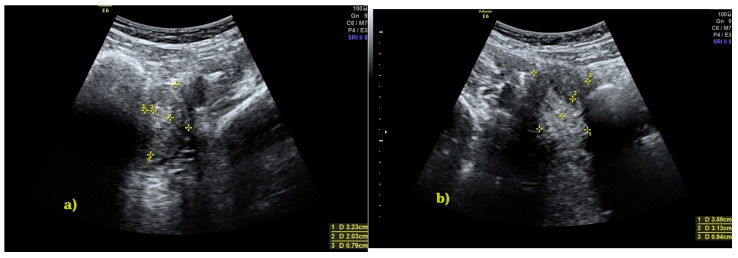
Transabdominal ultrasound showing two uterine bodies: (a) left uterine body, 32 × 20 mm; (b) right uterine body, 36 × 31 mm.
